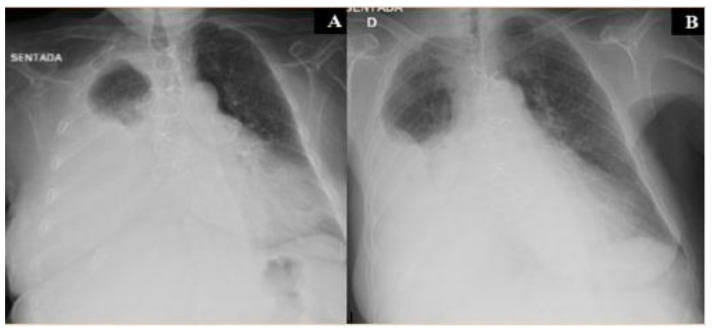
Thoracic X-Ray showing increased cardiac index and a large right pleural effusion, before thoracentesis (A); Thoracic X-Ray showing less prominent right pleural effusion, after thoracentesis (B).
